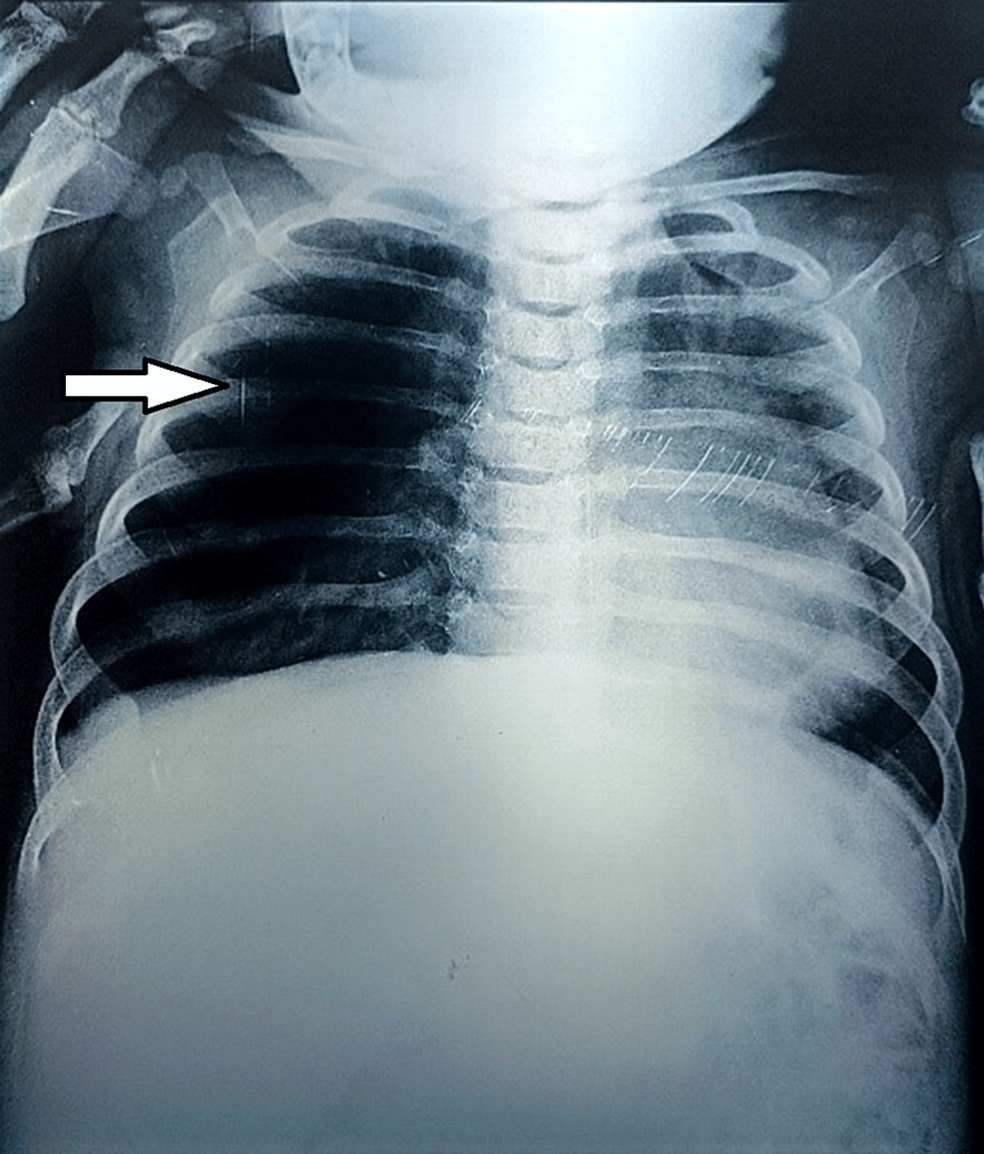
Chest x-ray of the second patient showing hyperlucent right hemithorax. Hyperlucent right hemithorax
